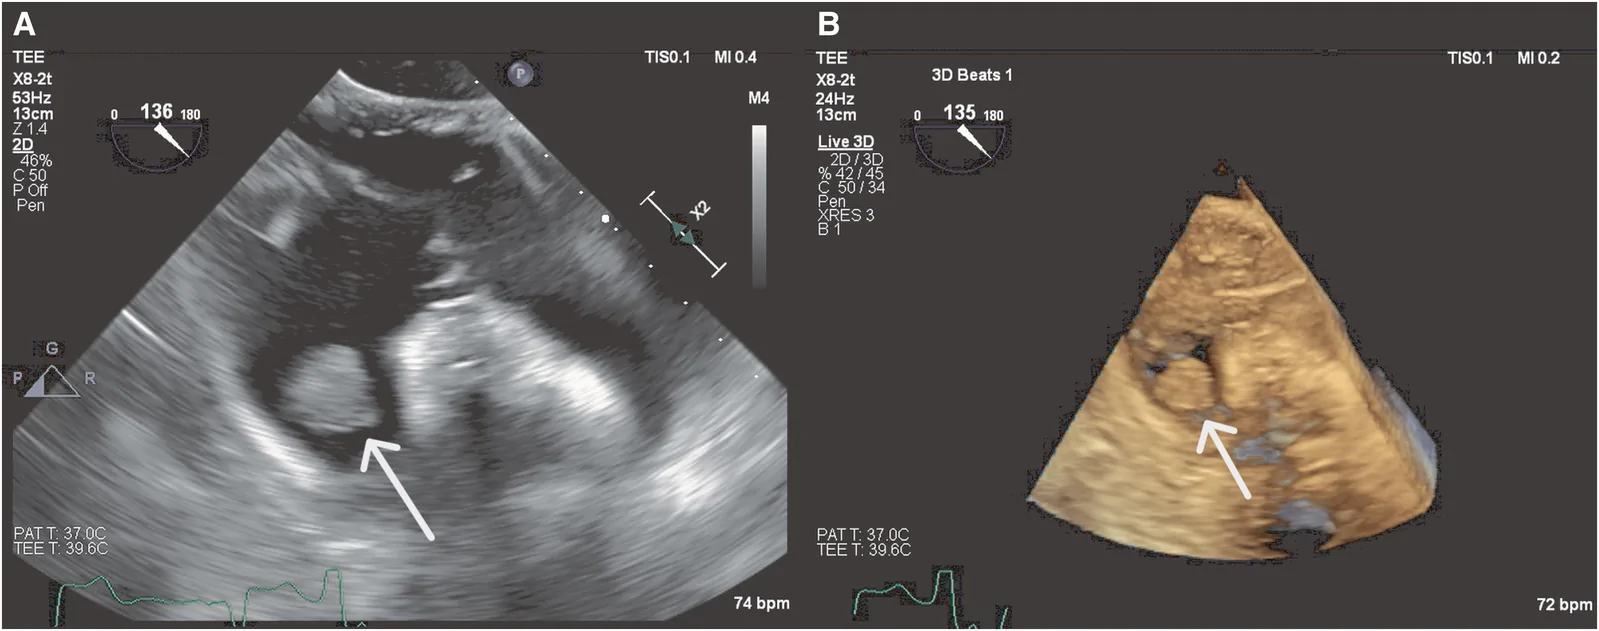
Transoesophageal echocardiography midesophageal views. (*A*) At 136°, a large echogenic mass measuring ∼3.0 × 2.4 cm is seen within the right ventricular outflow tract, along the right ventricular free wall in the subpulmonic region (white arrow). (*B*) A 3-dimensional view of the mass demonstrating endomyocardial invasion at the right ventricular free wall (white arrow).
